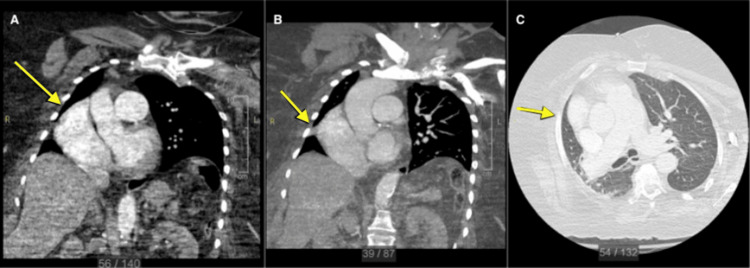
CT chest demonstrating isolated right middle lobe (RML) collapse and mediastinal displacement (A) Coronal CT demonstrating mediastinal shift, with the yellow arrow indicating rightward mediastinal displacement; (B) Coronal CT showing fissure displacement and RML volume loss, with the yellow arrow indicating the displaced fissure/RML volume loss; (C) Axial CT demonstrating triangular RML atelectasis, with the yellow arrow indicating the triangular atelectatic RML opacity.
